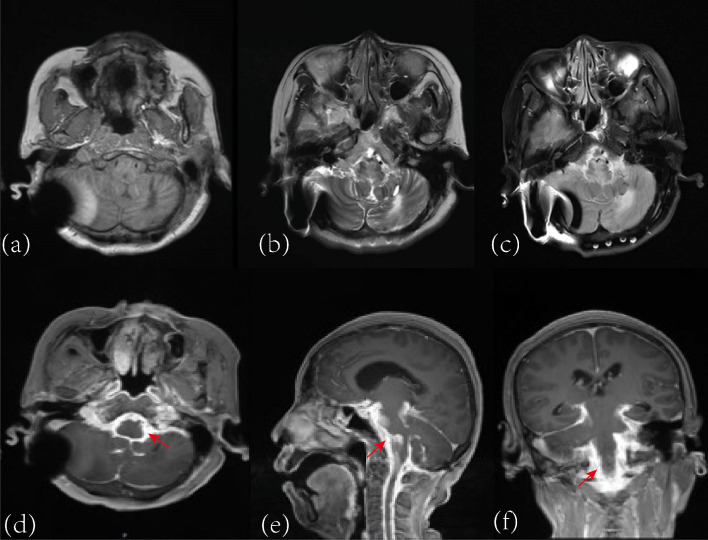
Brain MRI suggesting abnormal signals surrounding major arteries and some veins, potentially blocked the vascular. The plain scan sequence reveals patchy and linear long T1 (a) and long T2 signal (b) shadows in the bilateral cerebral arterial flow regions. High signal intensity is observed in the Flair sequence (c). The enhanced sequence displays multiple linear and patchy enhancement shadows surrounding the bilateral internal carotid arteries, vertebral arteries, cerebral arteries, and some veins [(d–f), red arrows]. The signal artifact in the right cerebellar region is caused by the ventriculoperitoneal shunt device placed in the right lateral ventricle.
